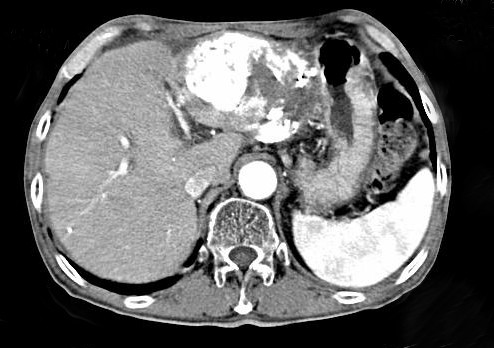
Enhanced CT scans after the combination treatment with TAE and stereotactic radiosurgery. The tumor size and vascularity improved, along with the significant reduction of the tumor markers (AFP and PIVKA-II). The serum level of AFP and PIVKA-II decreased from 164.9 ng/ml and 3692 mAU/ml to 14.4 ng/ml and 32 mAU/ml, respectively.
